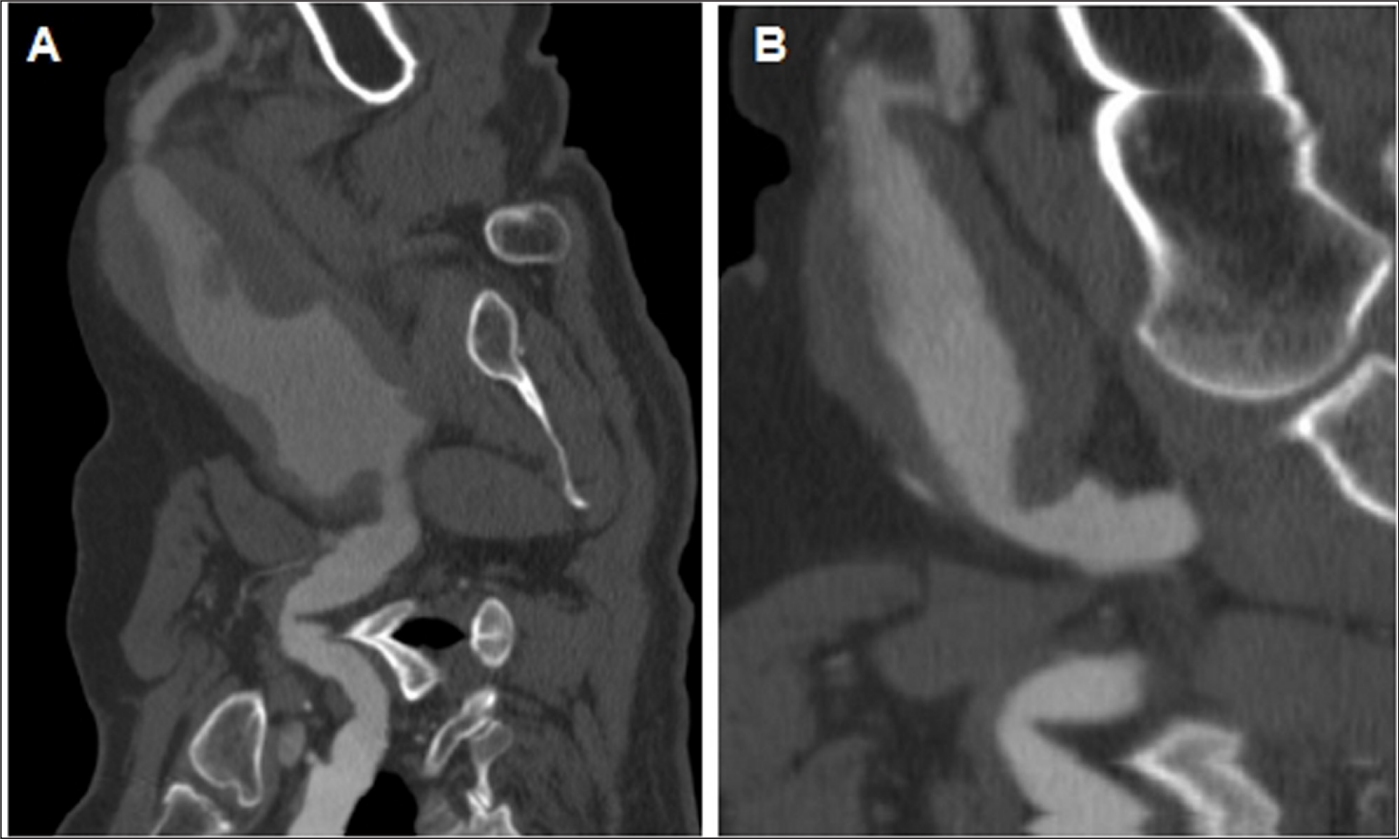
On a CT angiogram, bilateral axillary artery aneurysms were detected on curvilinear reformatted sections. (A) right, (B) Left.
